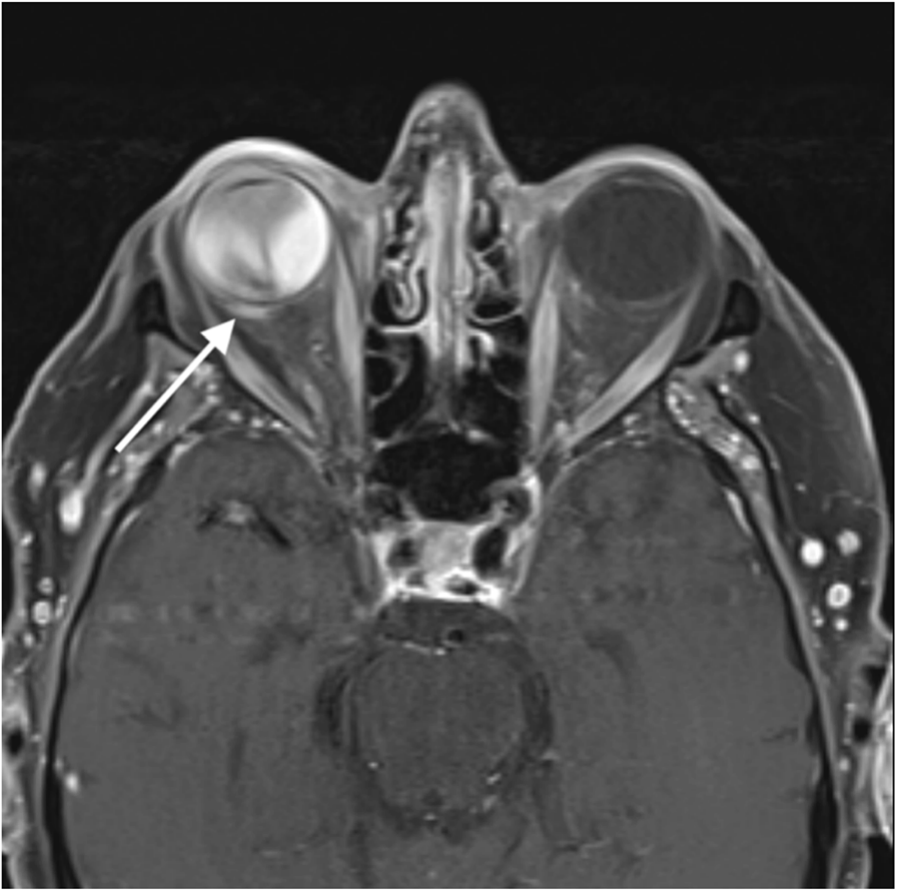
Axial post-contrast MRI orbits showing what appears to be contrast enhancement extending into the immediately adjacent retro-bulbar fat (white arrow), suspicious for extrascleral invasion
